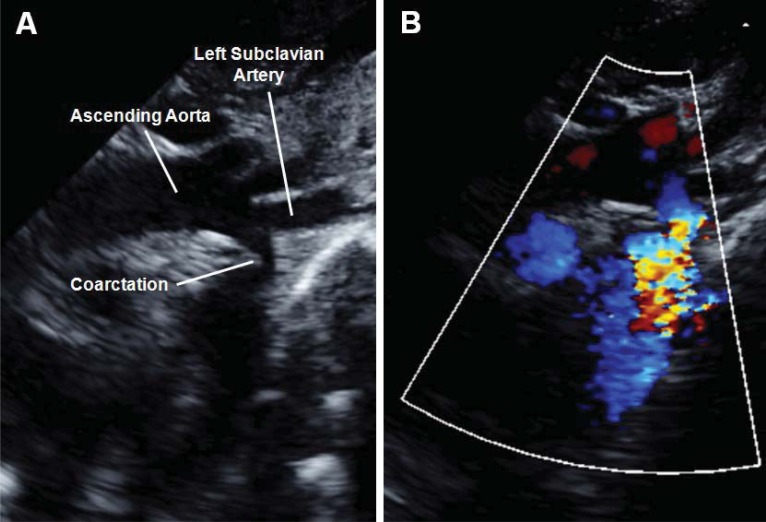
Panel A shows a two-dimensional transthoracic echocardiogram from the suprasternal view demonstrating a discrete narrowing just
